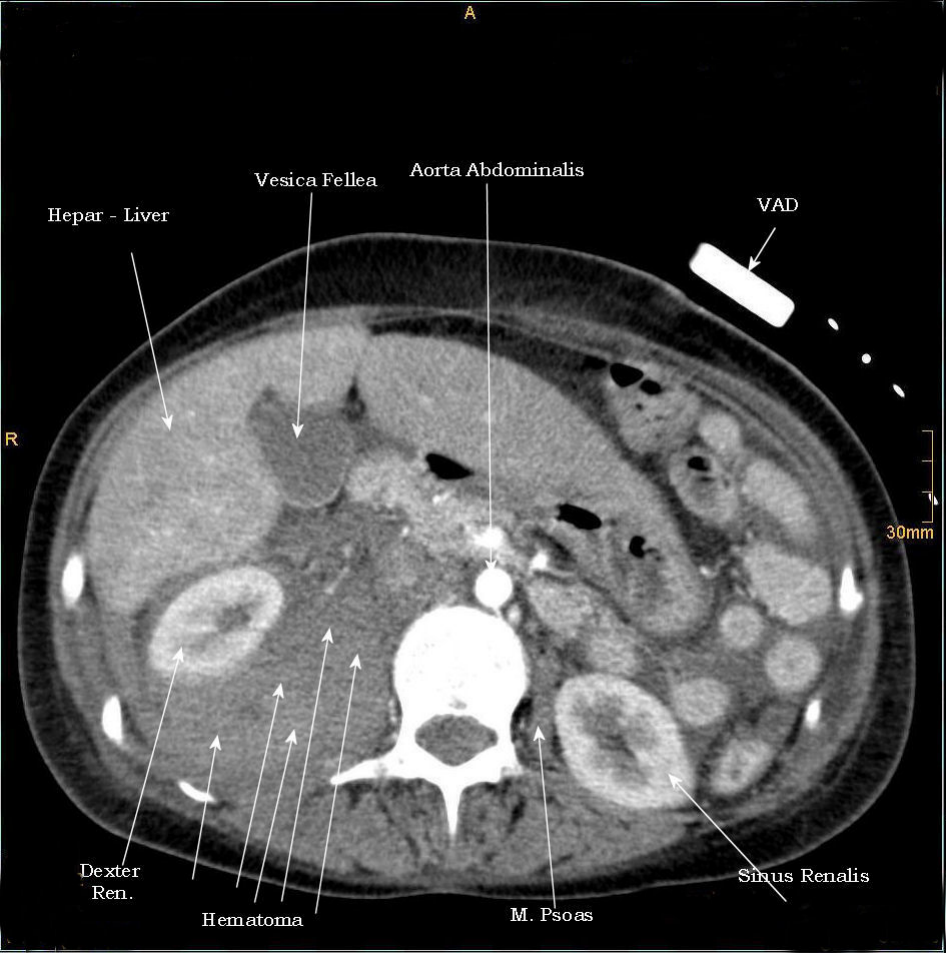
1^st ^case. CT - axial plan demonstrating a retroperitoneal hematoma adherent to the right psoas muscle, shifting the right renal lateral.
